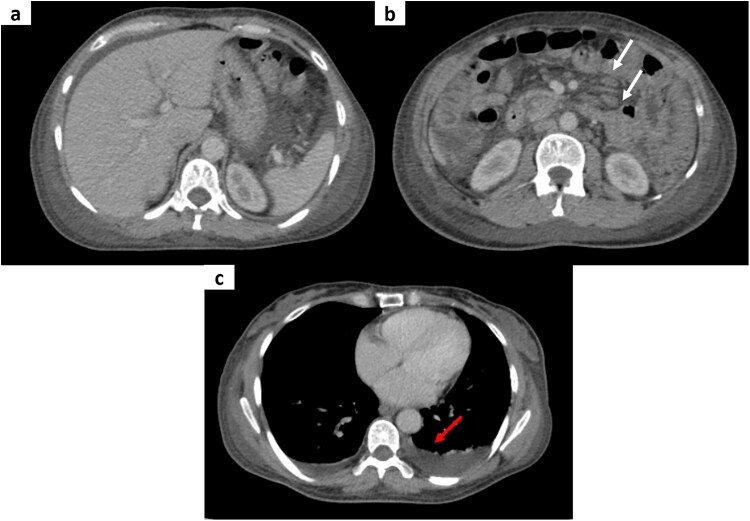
Axial contrast-enhanced CT images of the abdomen and pelvis in the portal phase (a–c) demonstrating extensive ascites with associated stranding and infiltration of the peritoneal fat, along with edematous changes in the subcutaneous tissues. Multiple homogeneous mesenteric lymph nodes are visible (white arrows). Bilateral pleural effusions are also present (red arrow).
